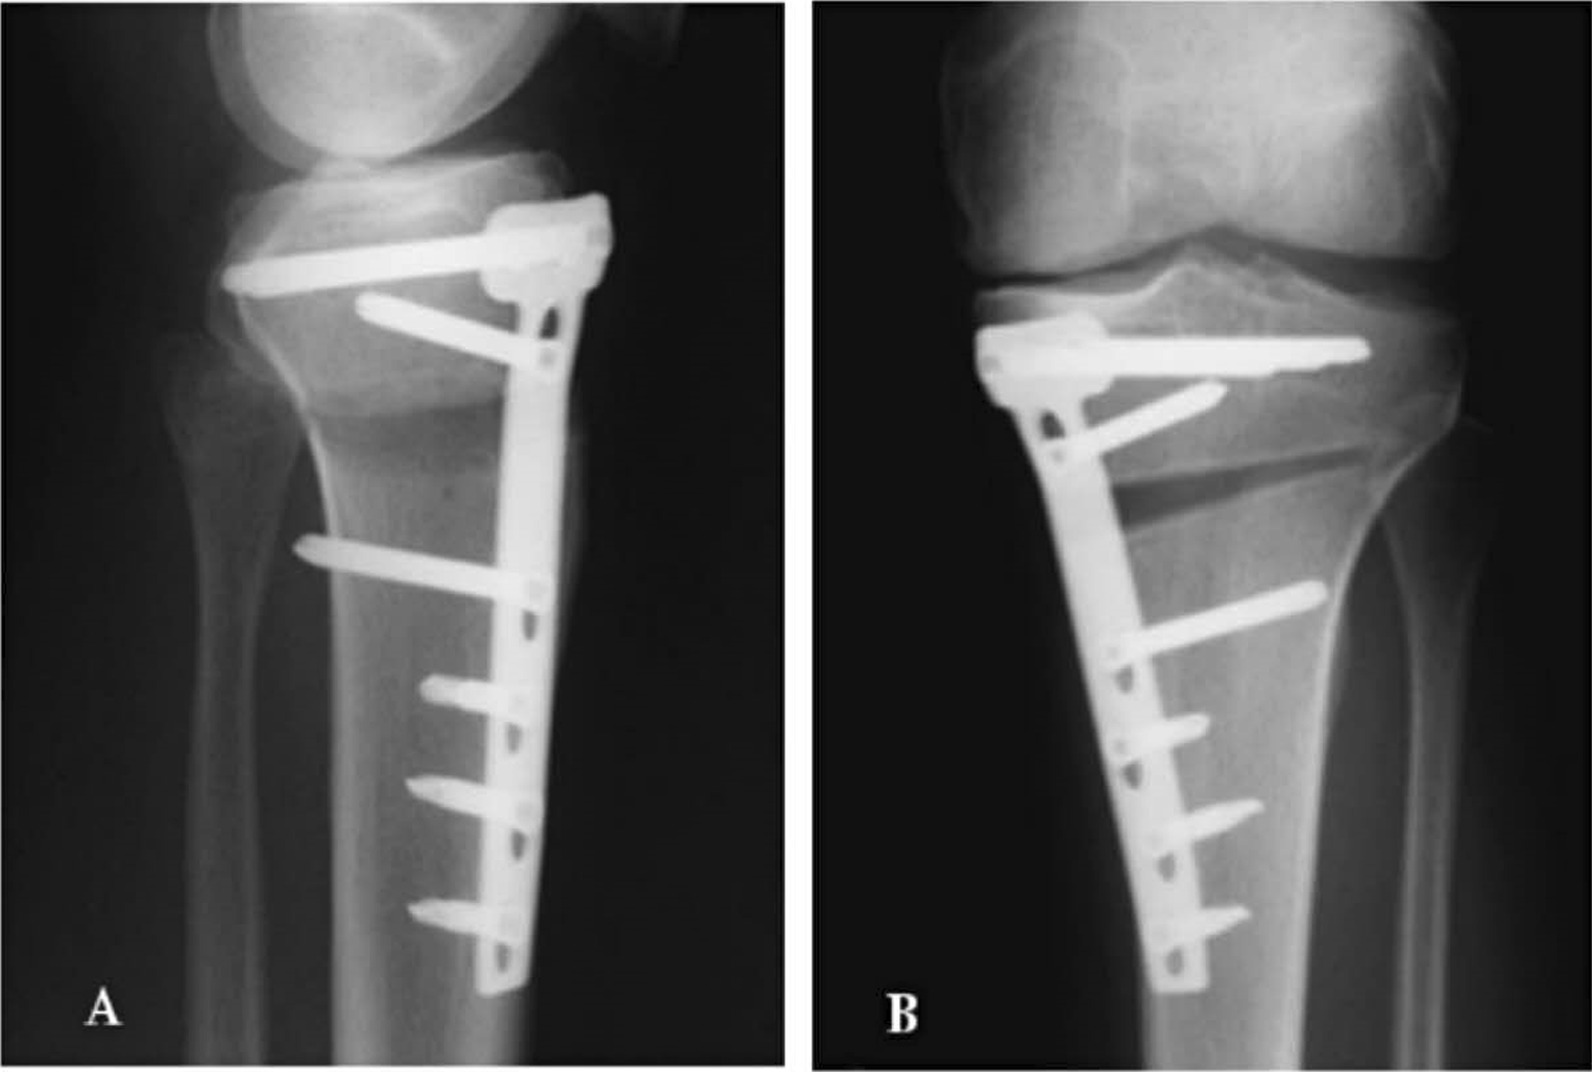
The lateral A and antero-posterior B X-ray of opening-wedge valgus HTO
